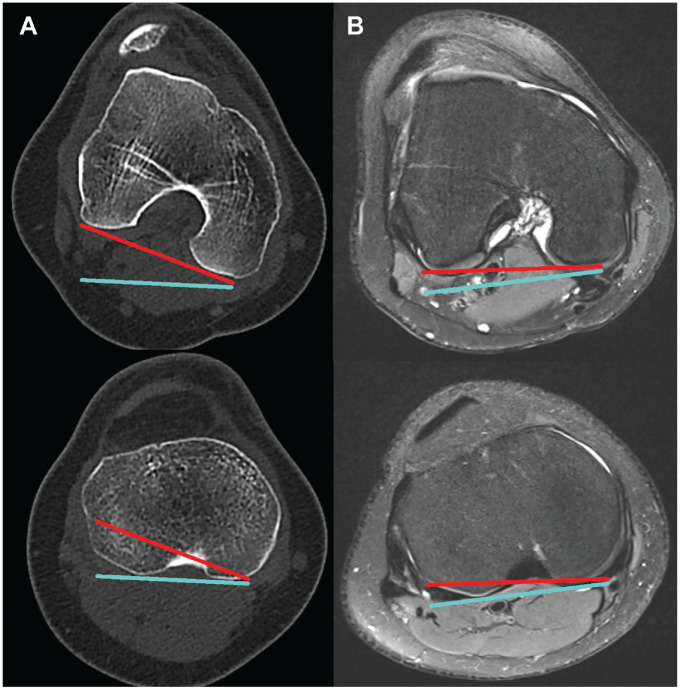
Measurement of tibiofemoral rotation in (A) CT and (B) MRI. The tangent line on the posterior femoral condyles is visualized in red. The tangent line on the posterior tibial condyles is visualized in turquoise. The tibiofemoral rotation is measured as the angle between the red and turquoise lines. CT, computed tomography; MRI, magnetic resonance imaging.
